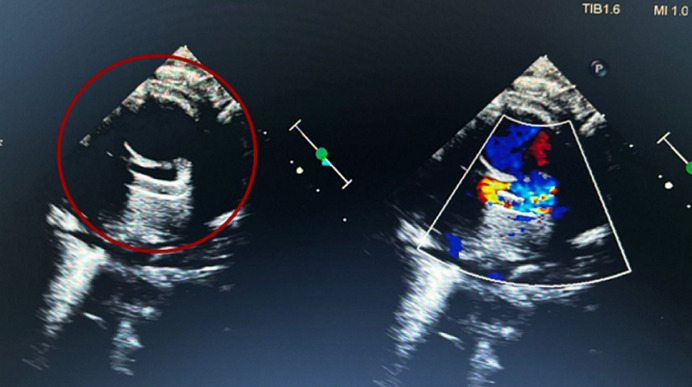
left side on echocardiogram displays the patent ductus arteriosus stenting; the right side shows the flow after the stent placement
